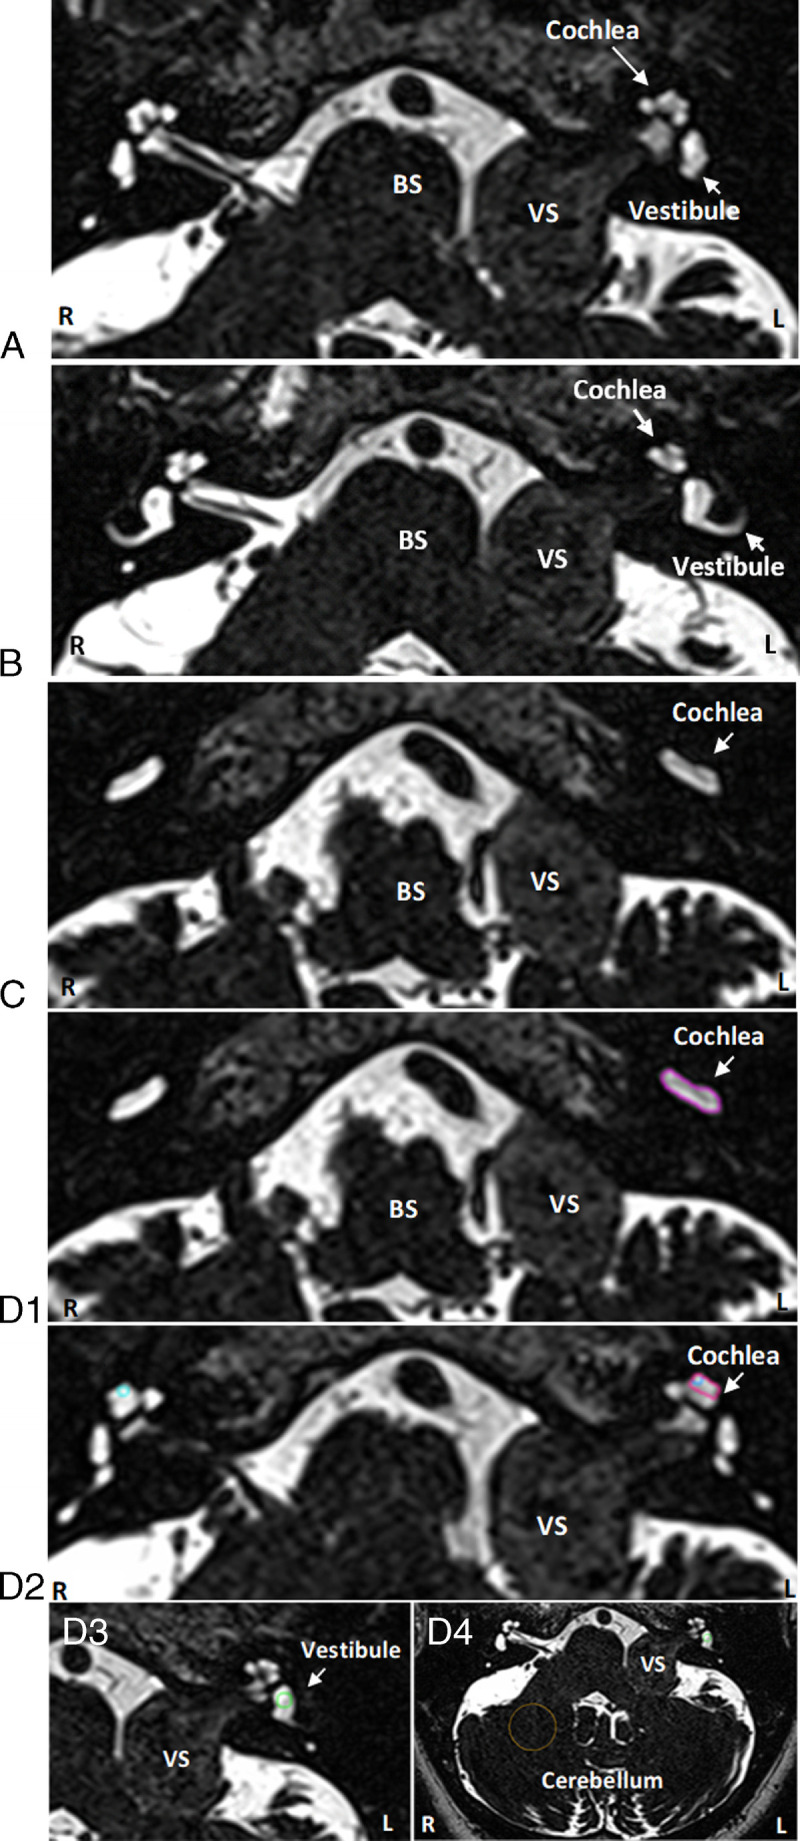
Axial T2-weighted 3D cisternogram MRI at different levels at the cerebellopontine angle from a patient with a left vestibular schwannoma demonstrates decreased signal intensity of the labyrinth on the tumor side. *A*, Decreased signal intensity of the left cochlea compared with the right cochlea, *B* includes a larger part of the vestibules and a semi-circular canal, and *C* basal turns of the cochleae. *D*, 1–4 show delineations of 1) larger basal cochlea region, 2) apical cochlea region, 3) vestibular region, and 4) cerebellar region (delineations in color in the online version). All images are from one patient. BS indicates brain stem; MRI, magnetic resonance imaging; VS, vestibular schwannoma.
